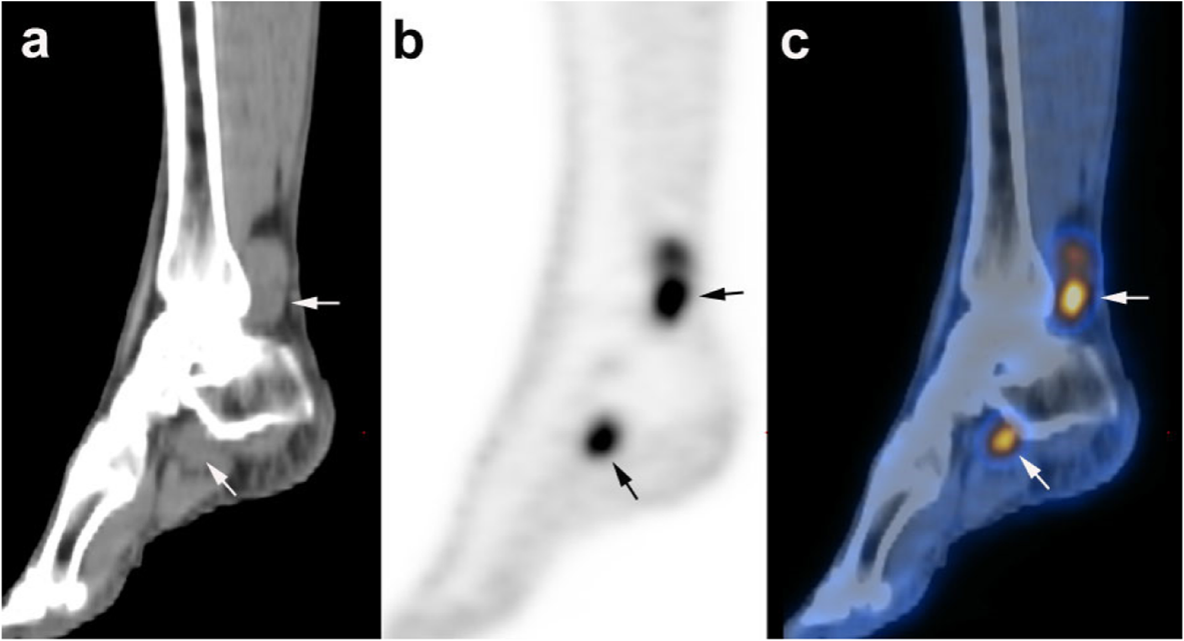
Sagittal positron emission tomography (PET; a), computed tomography (CT; b), and fused Fluorine-18 fluorodeoxyglucose (^18^F-FDG) PET/CT (c) of the patient’s left ankle and left foot. Two ^18^F-FDG-avid lesions were present in these locations beside the flexor hallucis longus muscle and tendon (arrows)
